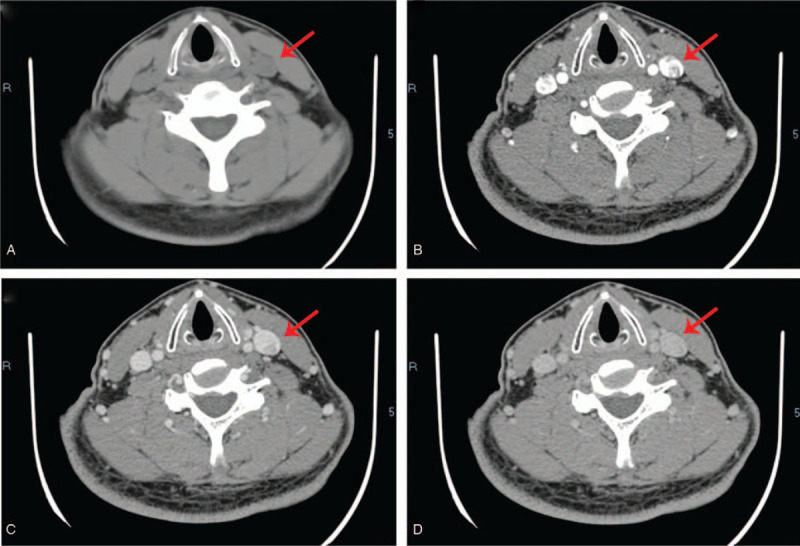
Enhanced CT of neck: (A) CT plain scan; (B) The image of enhanced CT arterial phase; (C) Venous phase; (D) Delayed phase. The red arrows mark the neoplasm.
